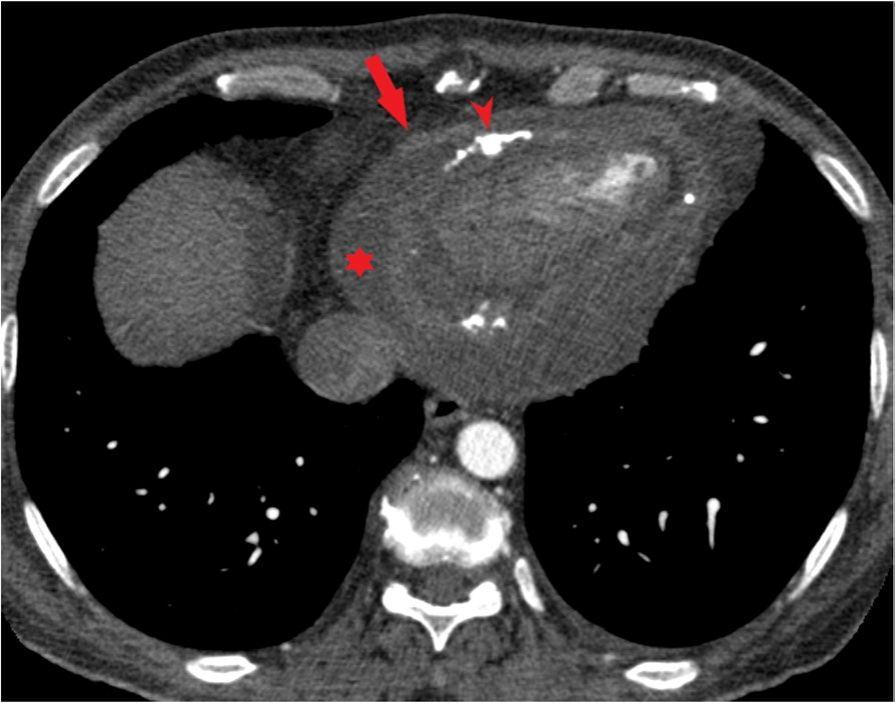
Contrast enhanced chest CT scan shows pericardial effusion (asterisk) with diffuse enhancing pericardial thickening (arrow). Areas of pericardial calcification are visible (arrowhead)
